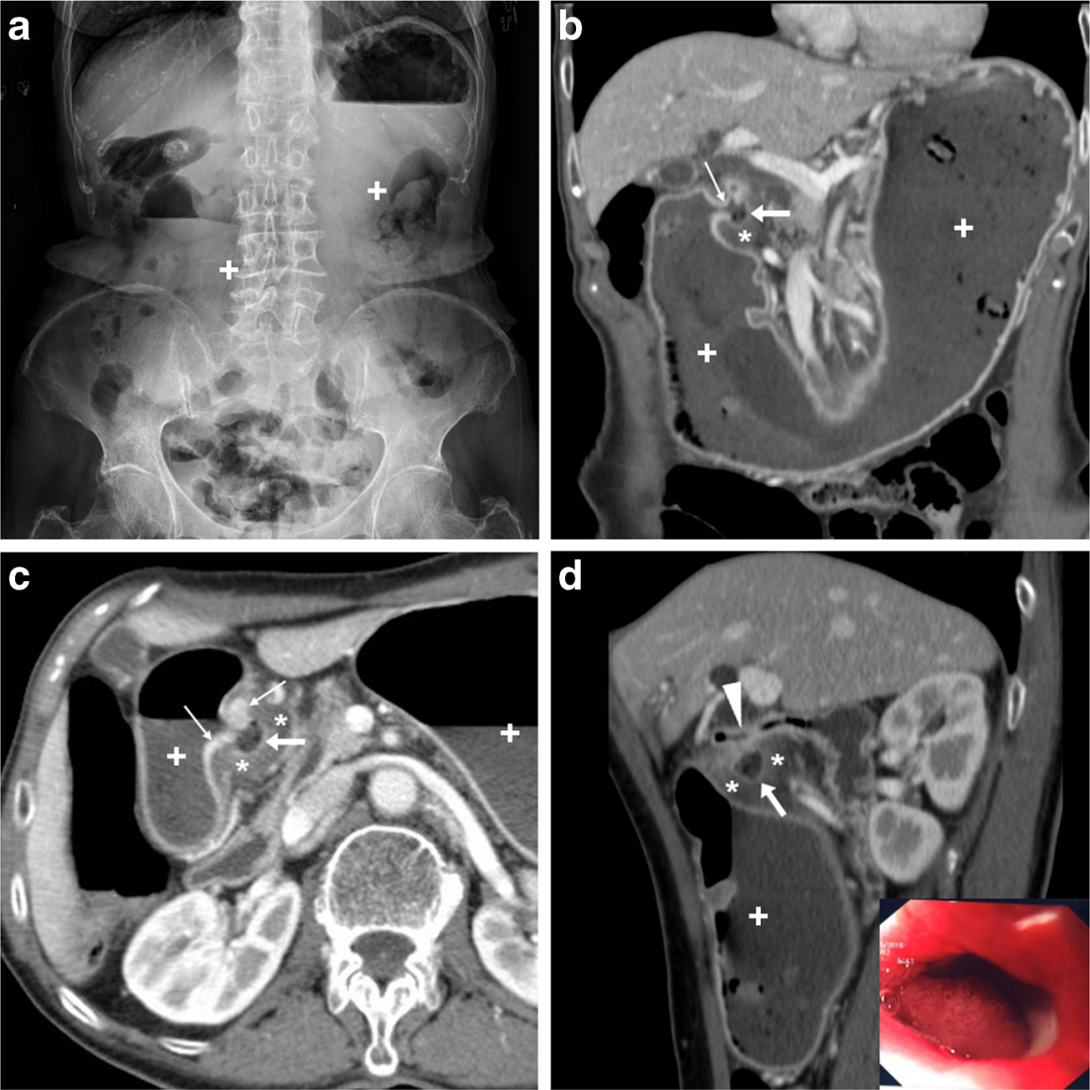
In a 68-year-old woman suffering from recurrent abdominal pain, nausea and 10-kg weight loss, abdominal radiographs (a) showed gastric overdistension by intraluminal stagnant fluid (+) despite fasting. Contrast-enhanced multidetector CT (b…d) confirmed overdistended stomach with normal mural thickness. The contracted pylorus and proximal duodenum showed circumferential hypoenhancing mural thickening (*) with mucosal hyperenhancement (thin arrows) focally interrupted at the site of an ulcer crater (arrows). Endoscopy confirmed moderate stricture of the proximal duodenum and post-pyloric ulcer (image in inset d) with fibrinous base, hyperaemic periphery, slightly irregular mucosal surface; biopsy excluded neoplastic changes. After PPI and anti-HP therapy, the patient ultimately required surgical treatment with duodenotomy, antrectomy and Roux-en-Y gastrojejunostomy [Adapted from Open Access ref. no [18]]
